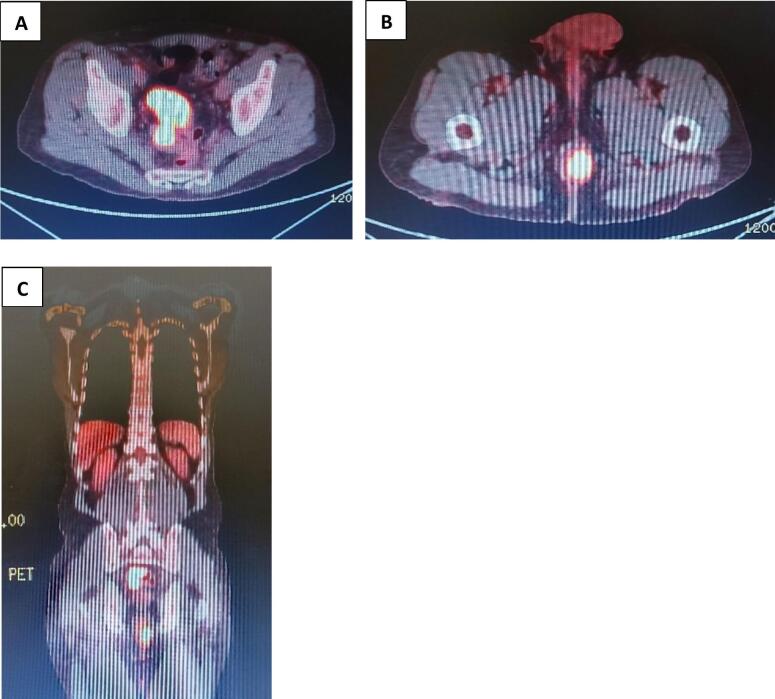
A. PET/CT scan, transversal view showing FDG avid soft tissue masse involving the sigmoid colon B. PET/CT scan, transversal view showing FDG avid soft tissue masse involving the anus C. PET/CT scan, coronal view showing FDG avid soft tissue masses involving the sigmoid colon as well as the anus.
